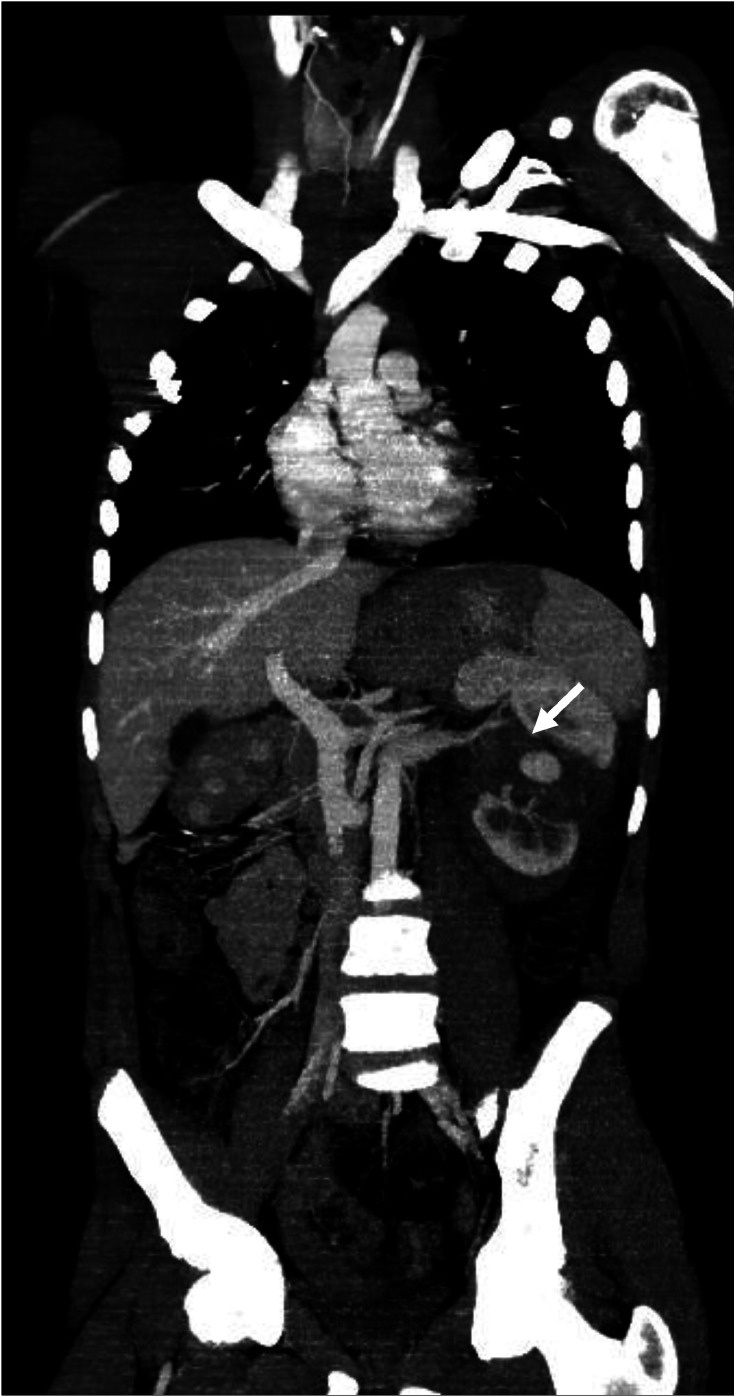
Coronal contrast-enhanced abdominal CT showing a renal laceration extending through the renal hilum with devascularization of the medial portion of the left kidney, involving the region from the lower to mid pole. The left renal artery is patent up to its division; beyond that point, it becomes difficult to delineate.
